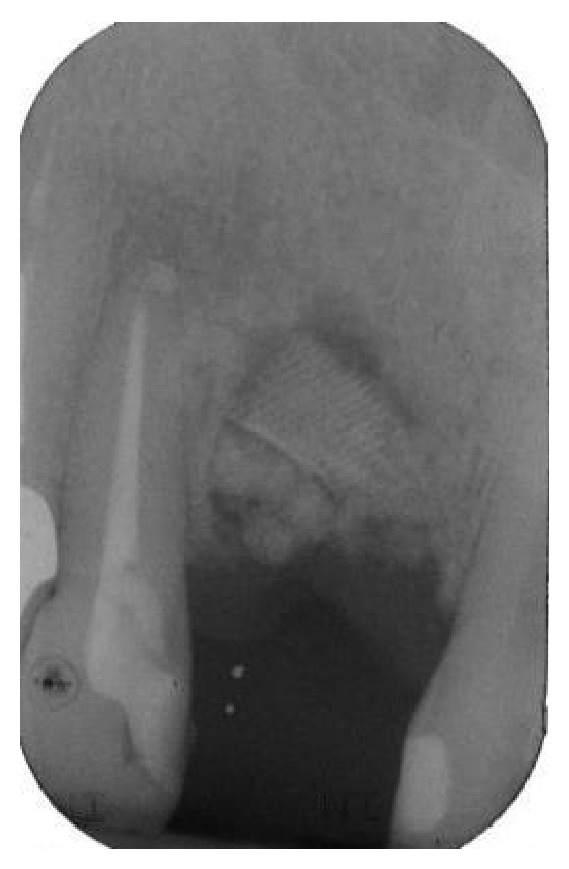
A postoperative periapical radiograph was taken to confirm the filling of the postextraction socket.
